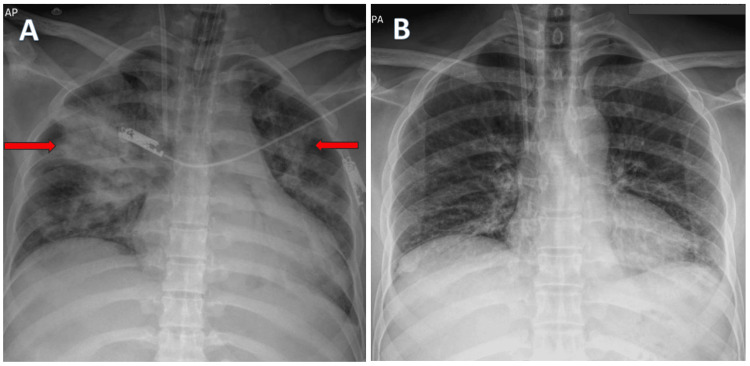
Serial chest radiographs showing pulmonary involvement and gradual radiological improvement following antibiotic therapy in severe scrub typhus. (A) Chest radiograph on admission showing bilateral patchy alveolar infiltrates (red arrows), consistent with scrub typhus pneumonitis. (B) Serial chest X-ray on day seven of hospitalization showing marked resolution of infiltrates, consistent with radiological improvement following antimicrobial therapy and supportive care.
